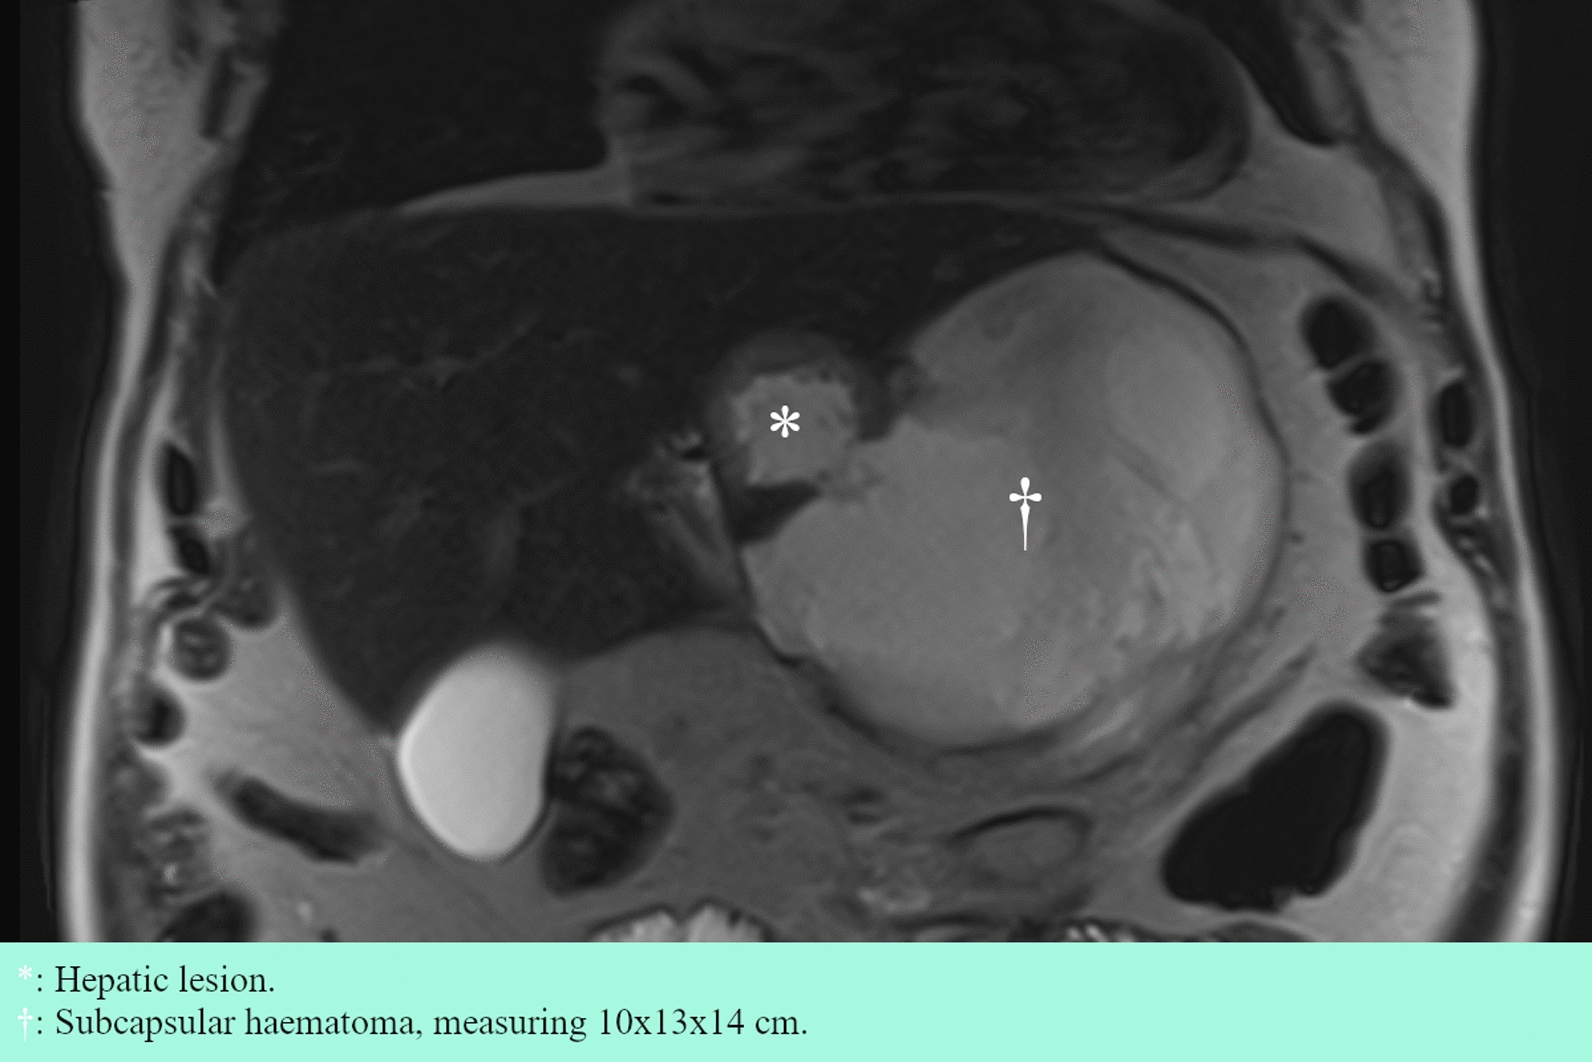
Coronal section of abdominal MRI, T2 weighted, 21 days after initial admission
